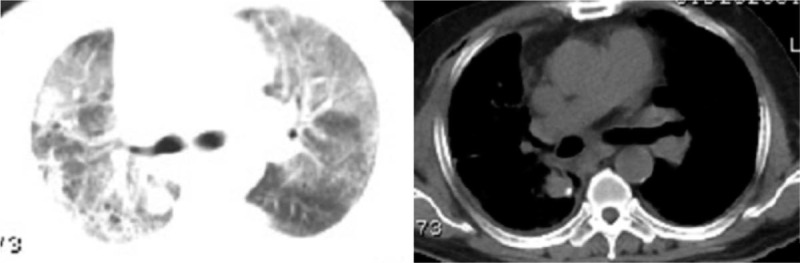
Chest computed tomography (CT) scan the fourth day after surgery: Multiple ground-glass opacities, consolidation, and patchy shadows on both lungs, bilateral pleural effusion with right-side encapsulated hydropneumothorax.
